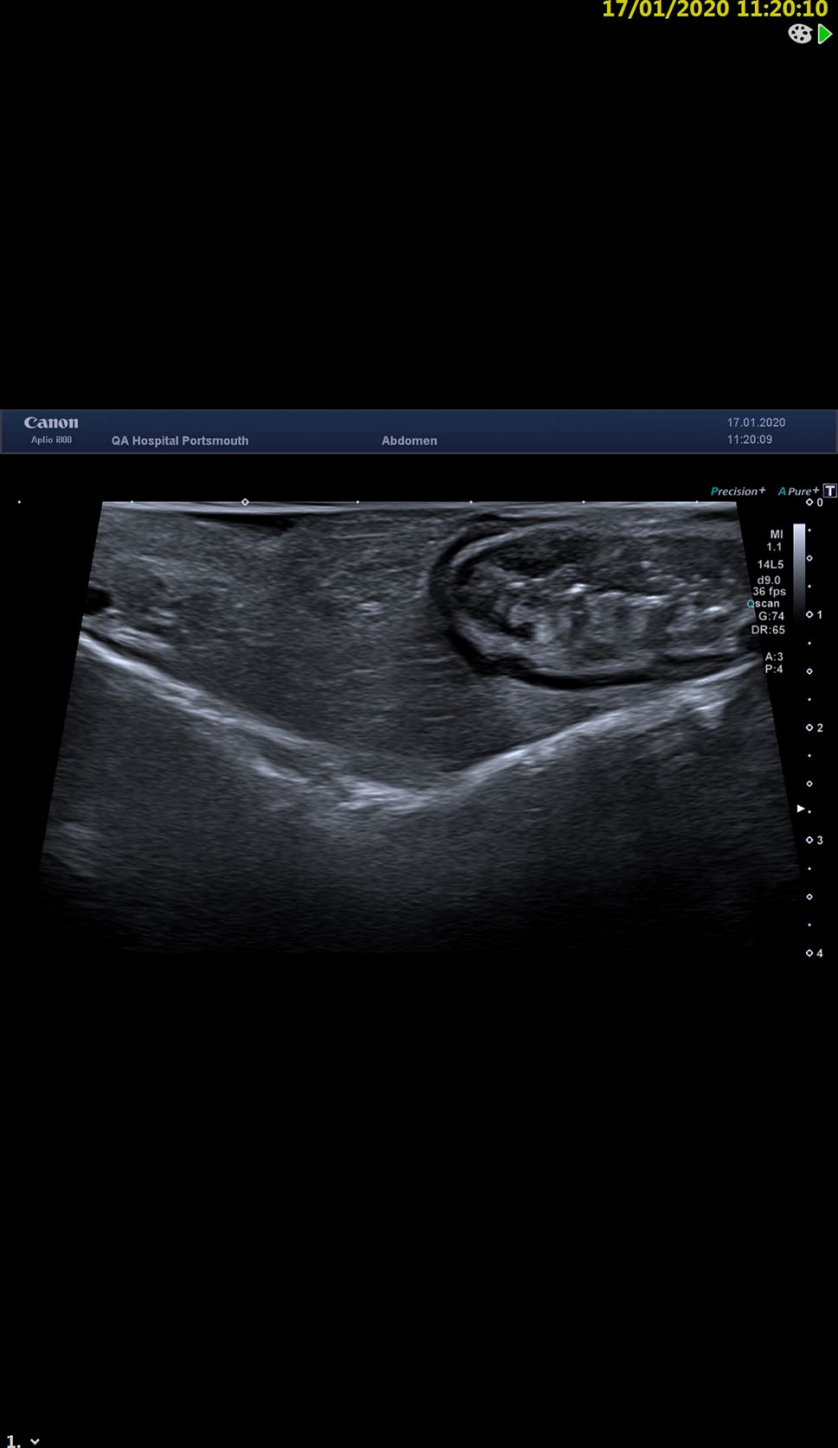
Mesenteric thickening on intraoperative ultrasound. *Thickening of the small bowel mesentery
